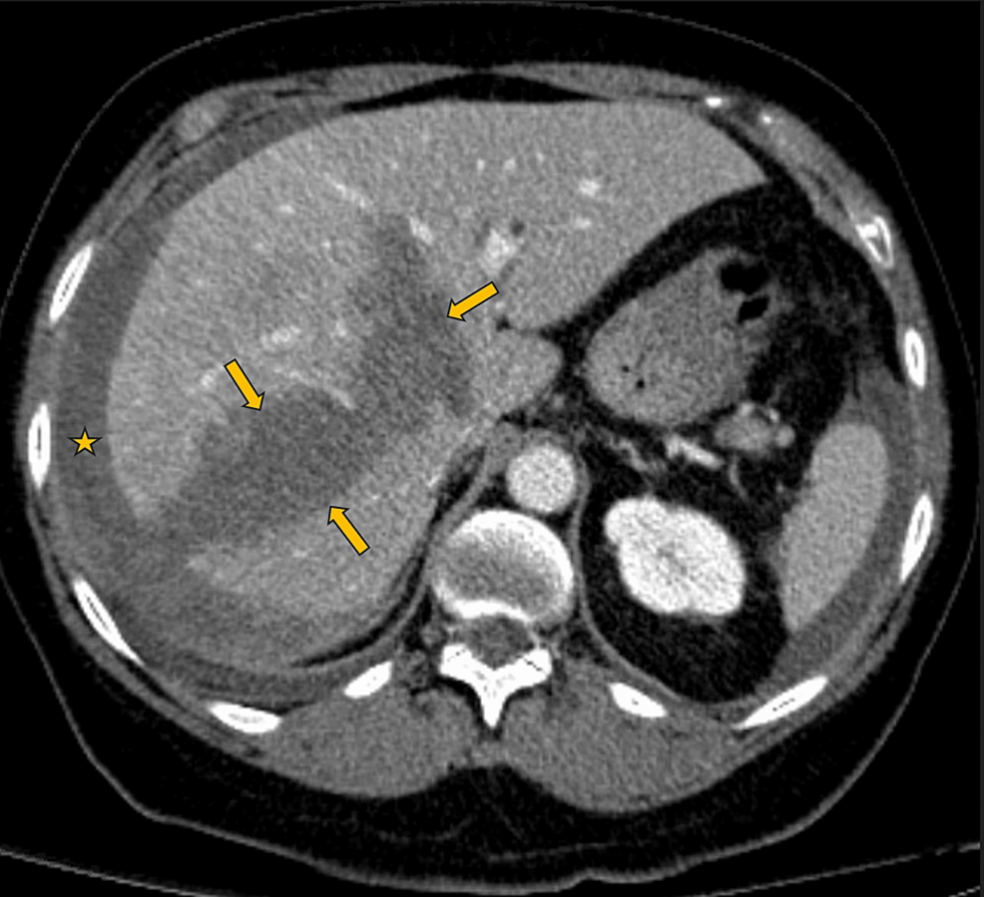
Hospital day 4: CT angiogram of abdomen and pelvis - venous phase Arrows - identify the edges of the hepatic laceration/hematoma; Star - extrahepatic hematoma
